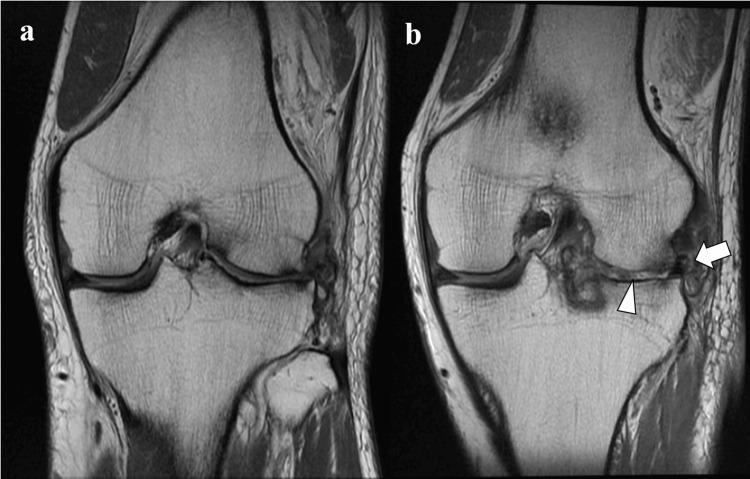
Coronal views of MRI (Case #8) a. Preoperative proton density-weighted image (TR2094, TE 30.46, FOV:160). b. Proton density-weighted image (TR2566, TE 22.87, FOV:160) 12 months postoperatively White arrow: meniscal allograft extrusion. Arrowhead: delaminated autologous chondrocyte transplantation MRI: magnetic resonance imaging
